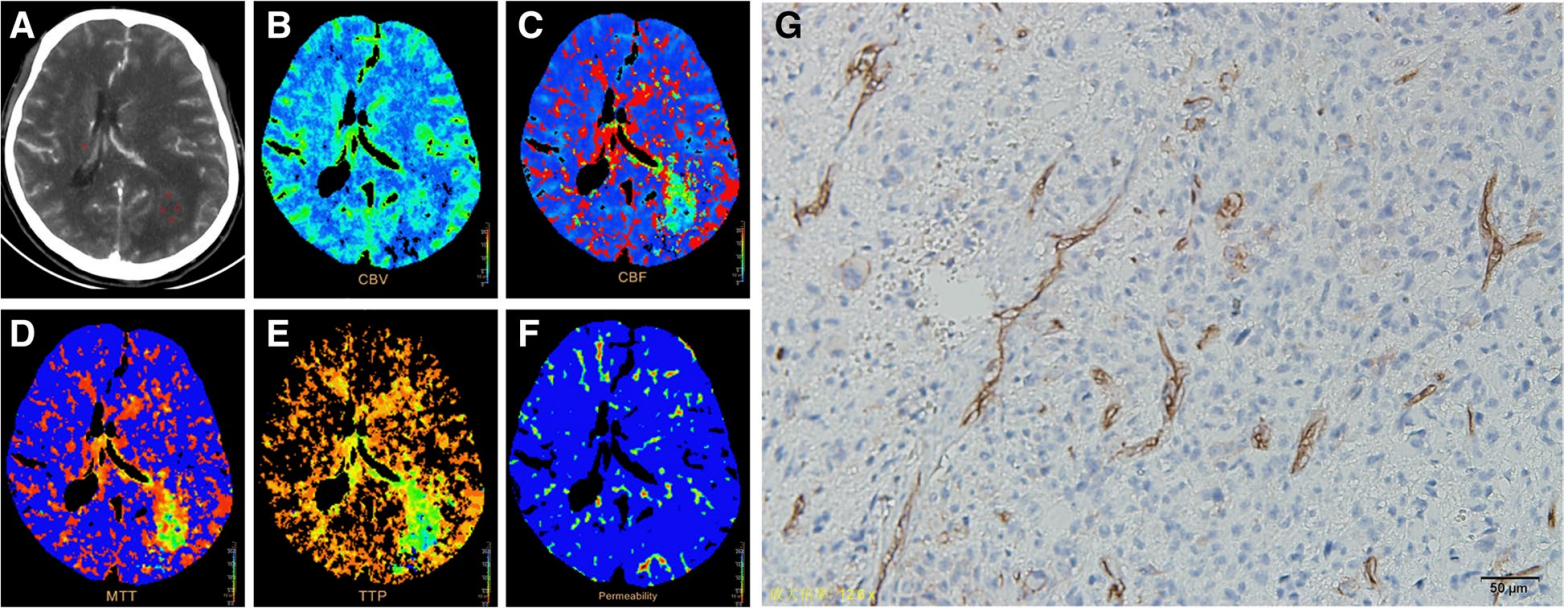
45-year-old man with Left frontal-parietal-occipital GBM, WHO grade IV, IDH-wt, TERT-mut, MGMT-met. A Transverse CT image and ROIs in red points. B PCT CBV map. C PCT CBF map. D MTT map. E TTP map. F PS map. E MVD map
